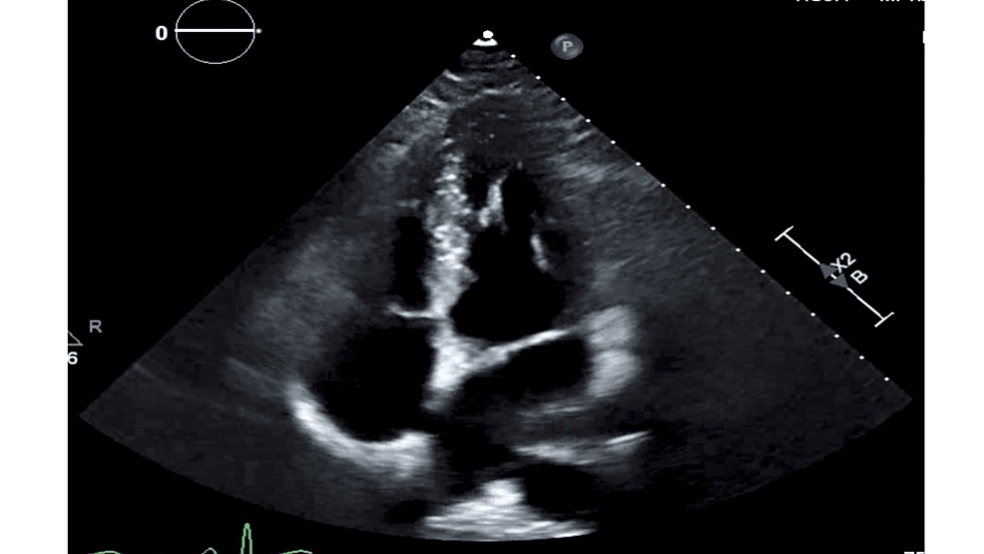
Echocardiogram done at presentation showing the communication between the right and left atria via the patent foramen ovale
